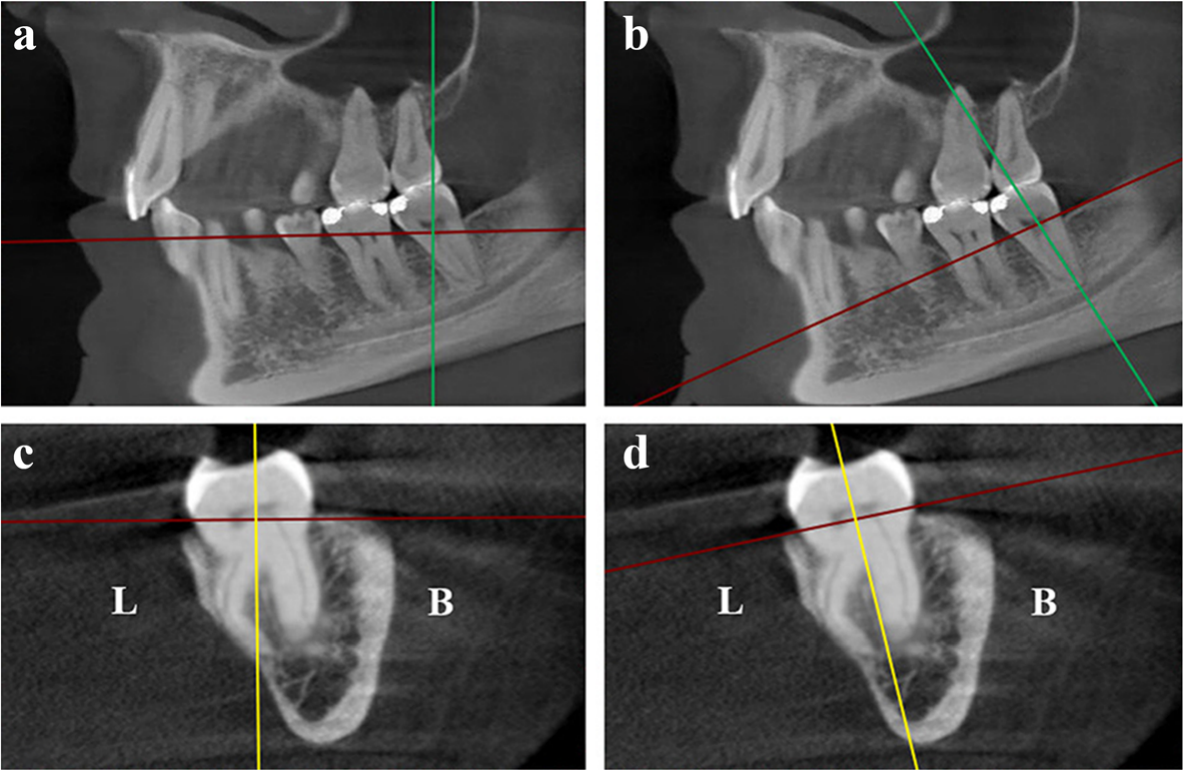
Cone-beam computed tomography. a Sagittal view. *Green line*: coronal axis. *Red line*: axial axis. The axes are incorrectly oriented with respect to tooth 3.7. b
